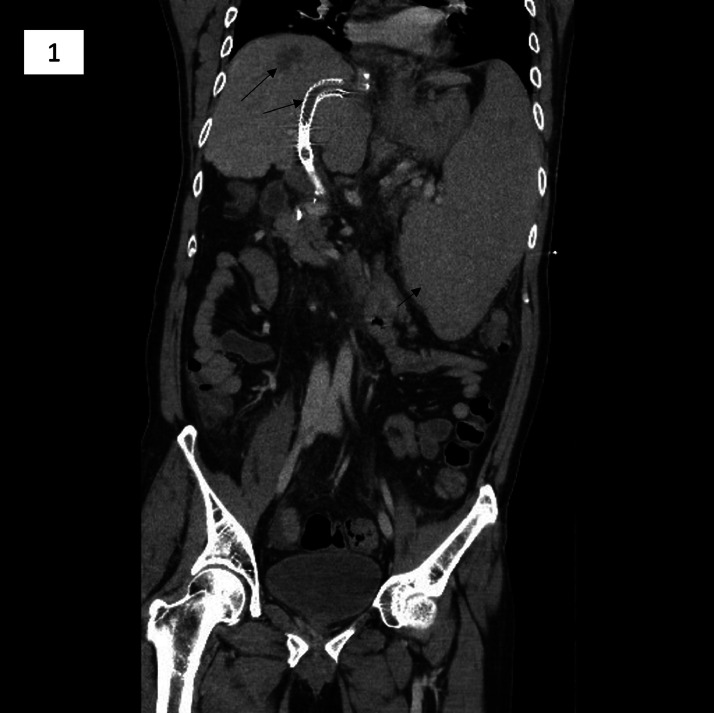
Coronal CT image shows thrombotic occlusion of the TIPS shunt and massive splenomegaly. Note hepatic dome hypodense lesion which represents post ablation changes related to a hepatocellular carcinoma.
